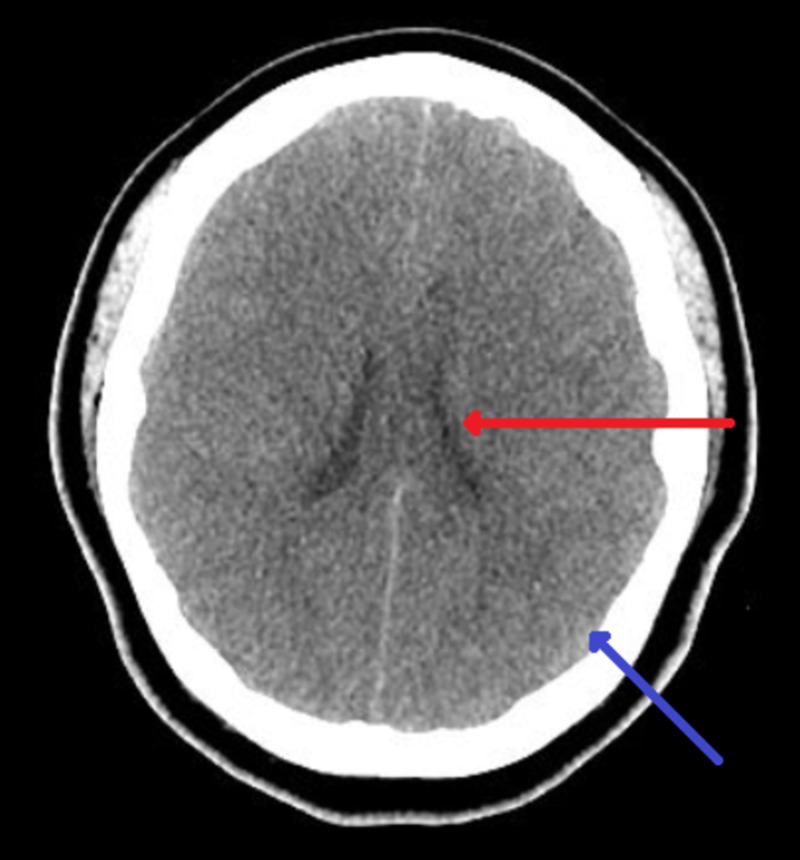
CT head showing the ventricles are slit like (red arrow) and the cortical sulci are diffusely absent (blue arrow), consistent with diffuse cerebral edema.
